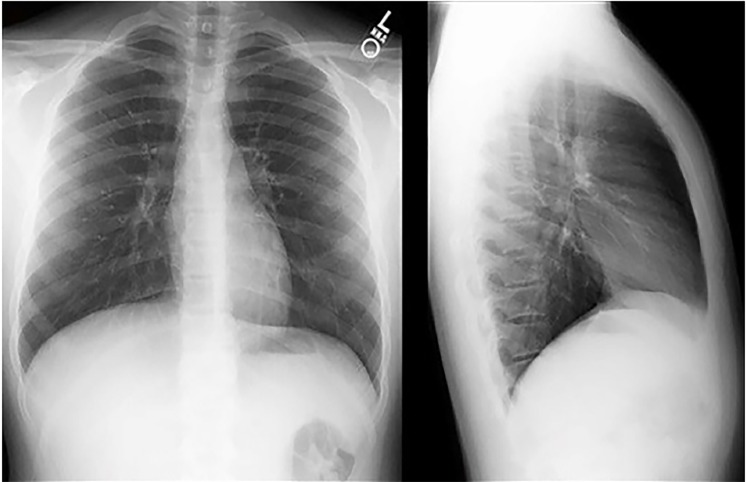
Chest radiograph of 83-year-old woman with fever and emesis.
